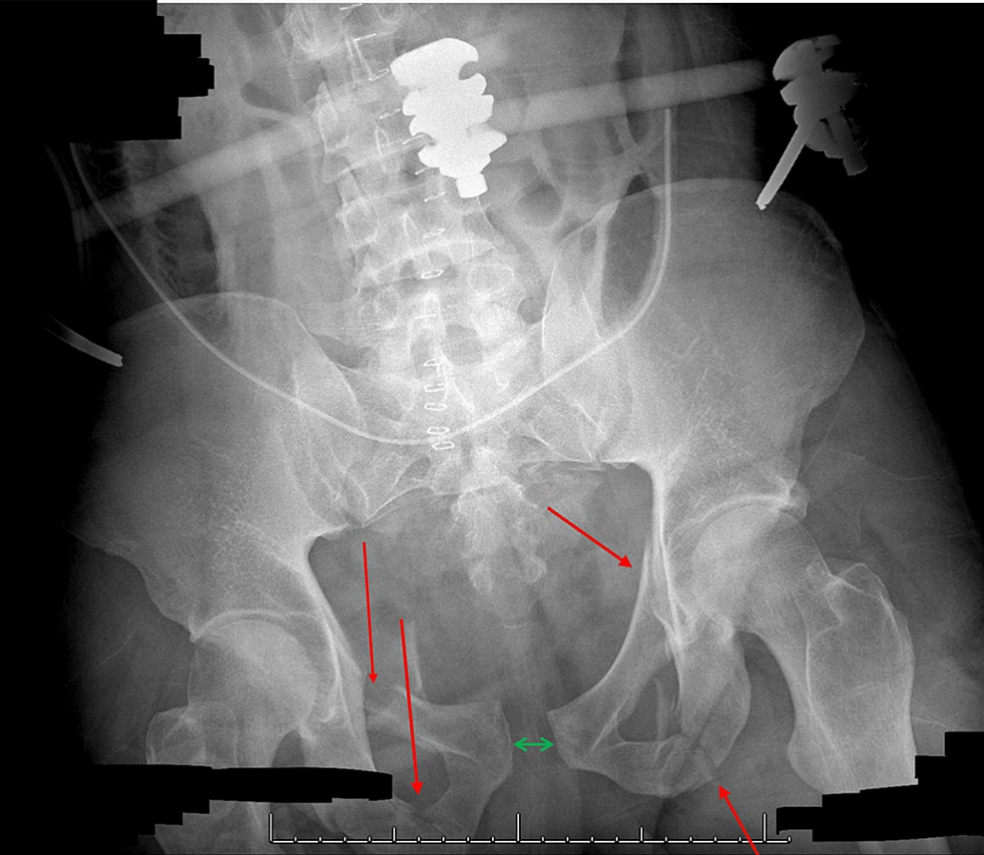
Pelvic X-ray of the external fixture before open-reduction internal fixation. Right superior and inferior pubic rami fractures are seen with minor displacement. There is a left superior pubic ramus fracture without significant displacement and a comminuted, mildly displaced fracture of the left inferior pubic ramus. Additionally, there is pubic symphysis diastasis (indicated by green line) and very mild widening of the sacroiliac joints. An external fixation device is noted. Points of fracture are highlighted by red arrows.
